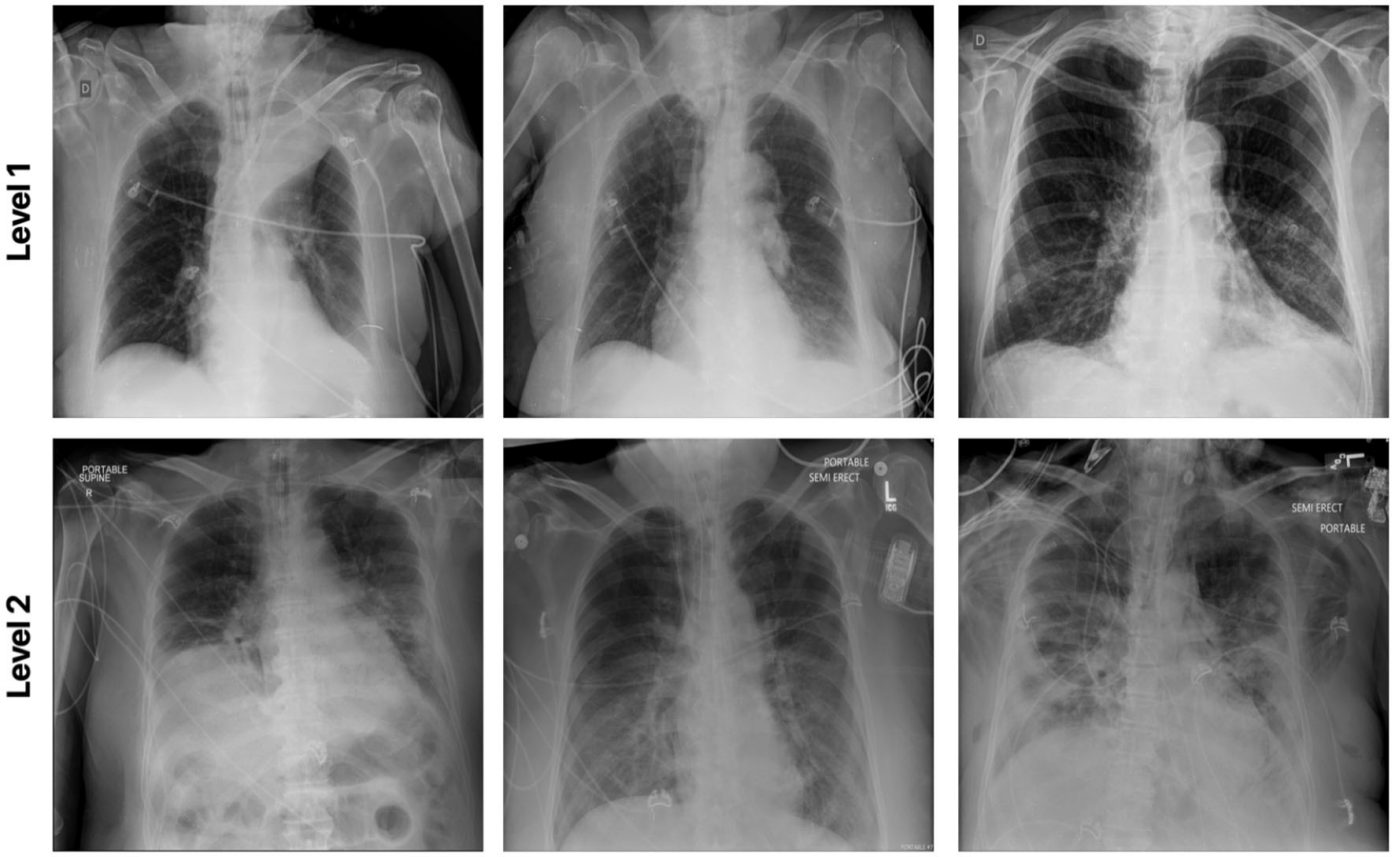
Example chest X-ray images from the RSNA RICORD COVID-19 Severity dataset.
